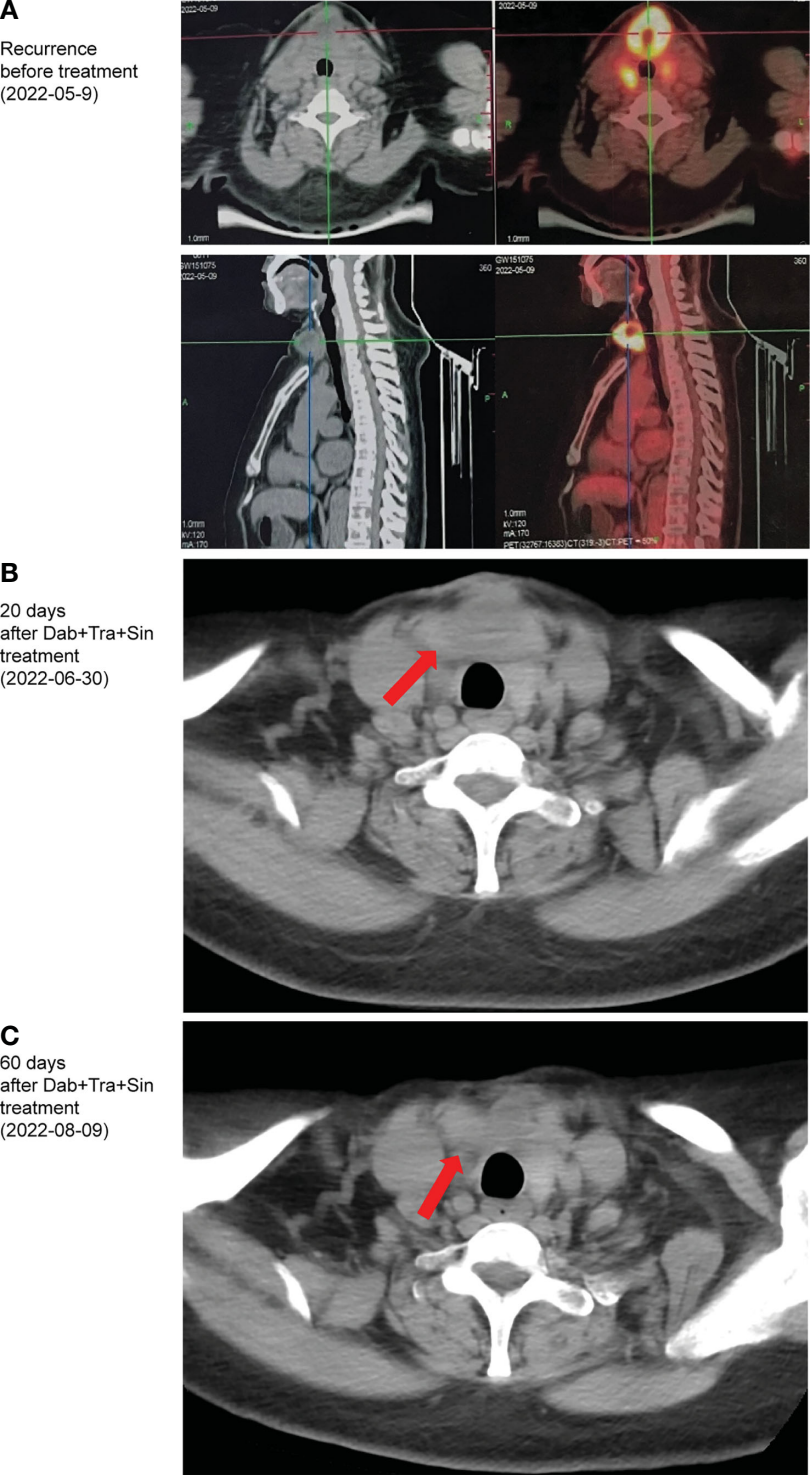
Computed tomography images showing the patient’ recurrence (A), as well as response to the triplet regime of sintilimab, dabrafenib, and trametinib after 20 (B), 60 (C) days of treatment.
